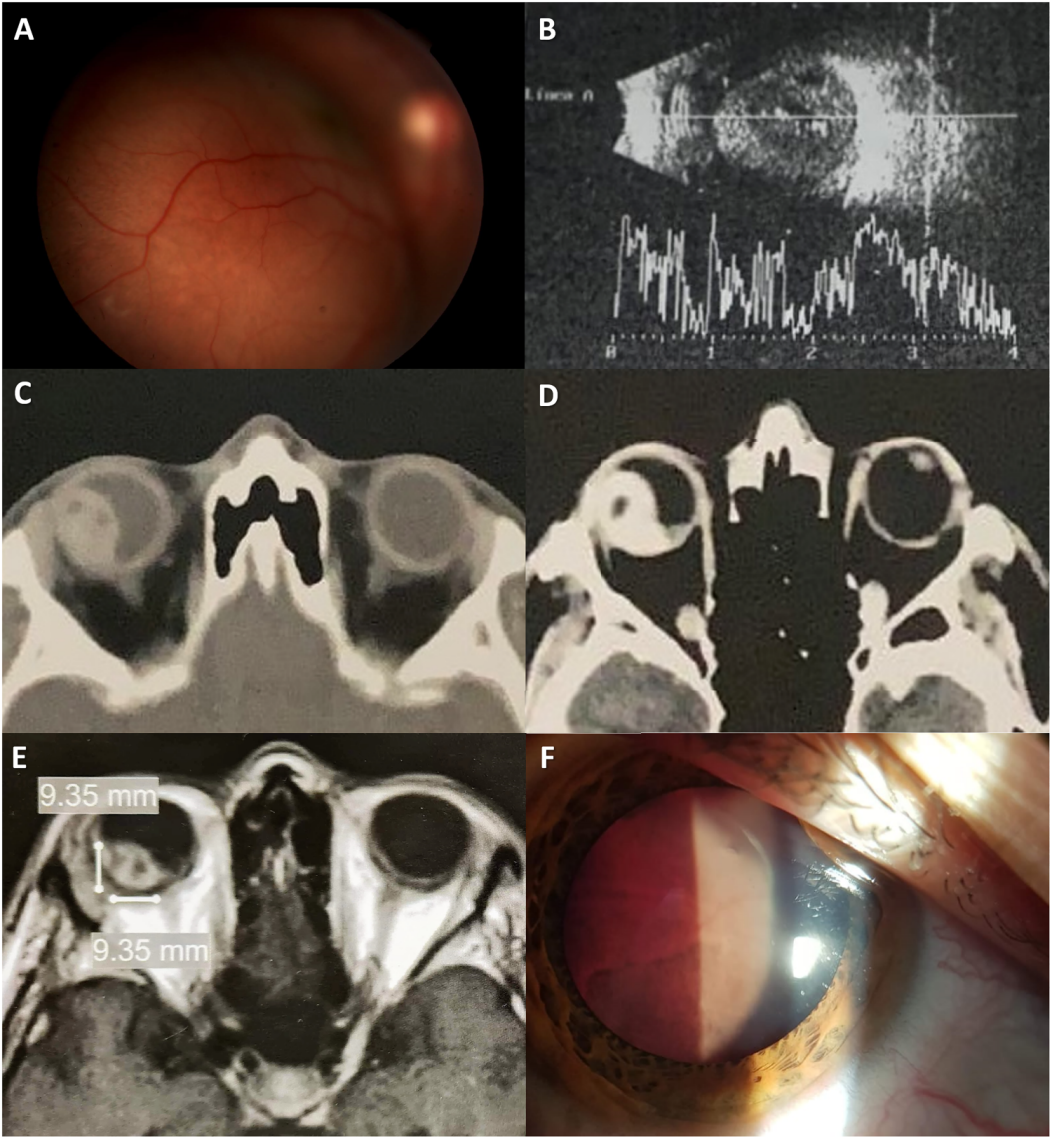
Multimodal evaluation of osteosarcoma choroidal metastasis. A) Right eye (OD) retinography, showing an elevated subretinal, pink, nodular mass on the posterior pole. B) A and B-scan OD ultrasound positive for a large, round, subretinal mass with irregular structure, medium/high overall reflectivity and areas of localized increased and decreased (probably necrosis-related air) reflectivity, with a height-to-base ratio of 1-1.2, approximately. C) Computerized tomography (CT) scan revealing a heterogeneous, well-defined mass within the uveal layer, hyperdense with areas of decreased density, and significant intraocular involvement. D) Contrast-enhanced CT showing a heterogeneously enhancing mass on OD, with areas of decreased and null enhancement. E) T1- weighted magnetic resonance imaging showing a well-defined, heterogeneous choroidal mass that appears isointense and has equal base and height. F) Anterior segment photography taken at a follow up visit showing a fully detached retina in contact with the posterior capsule of the lens.
